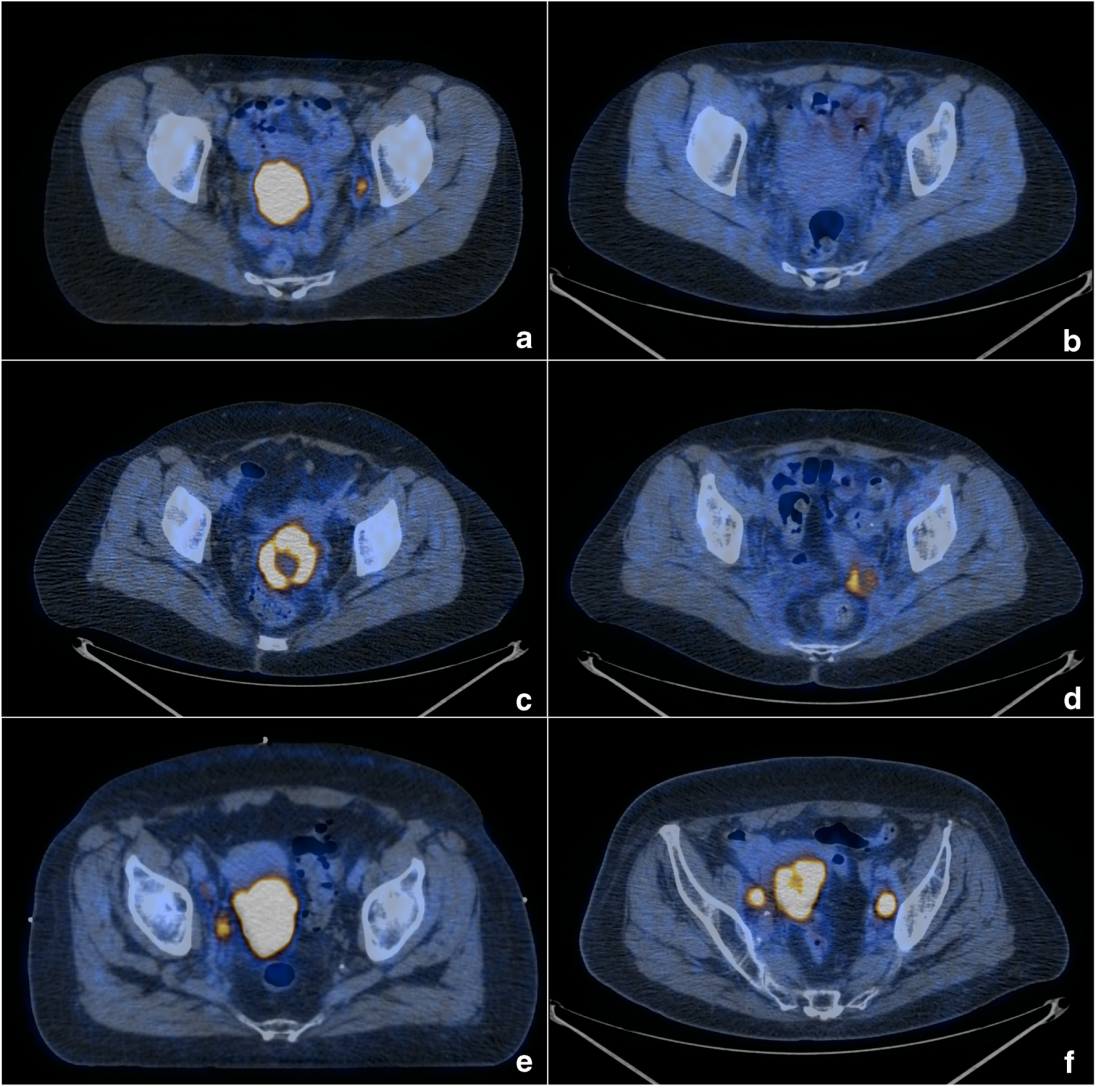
Axial PET/CT images before and after treatment: a, b showing a complete metabolic response; c, d showing a partial metabolic response; e, f showing progressive disease
